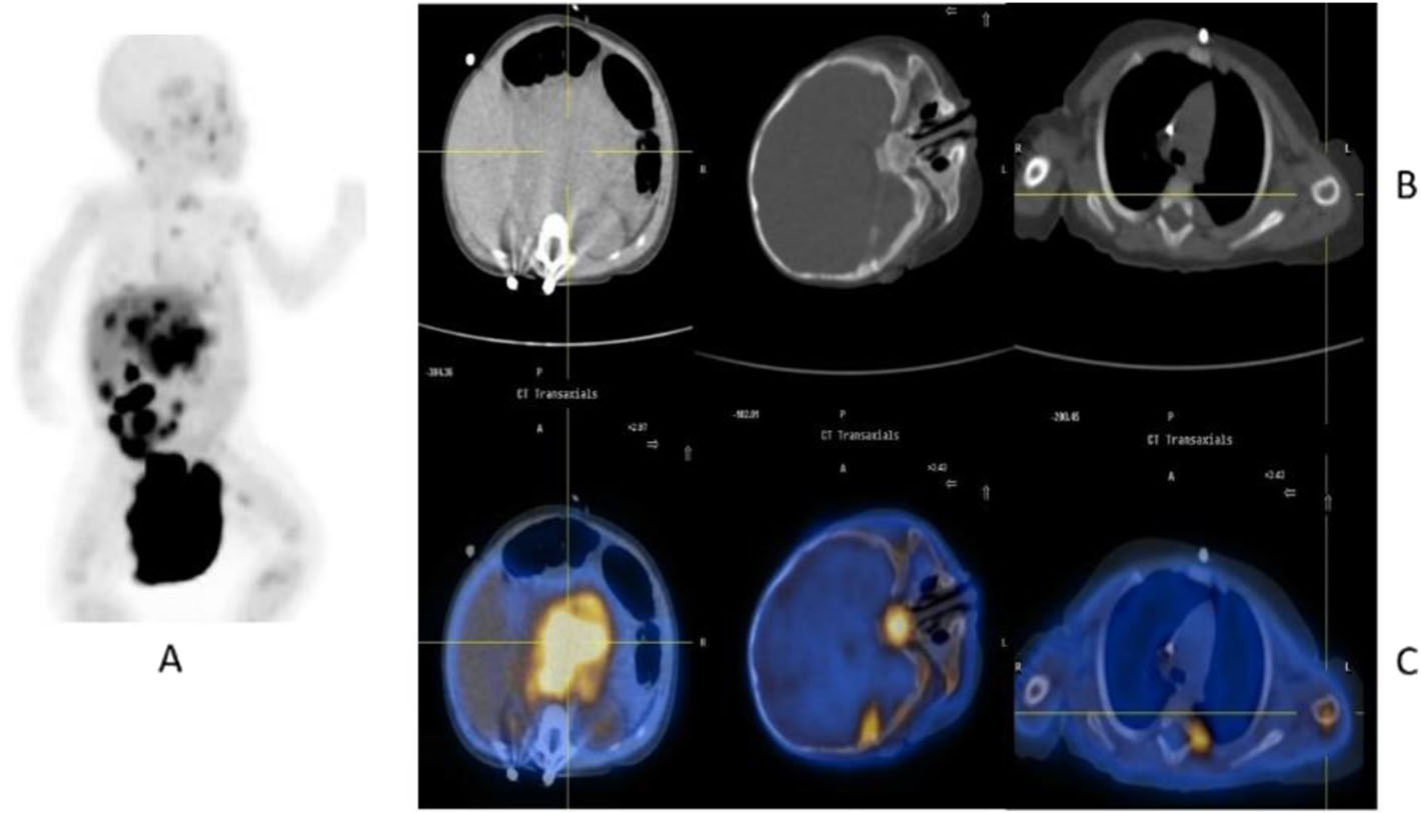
^18^F-FDOPA, neuroblastoma. *Clinical history*: 6 months old girl with neuroblastoma, stage IV at presentation. *PET/CT findings*: intense uptake of the tracer in multiple metastases in the liver and bones, in particular sphenoid and left humerus, seen on MIP (a), CT (b), and fused images (c)
